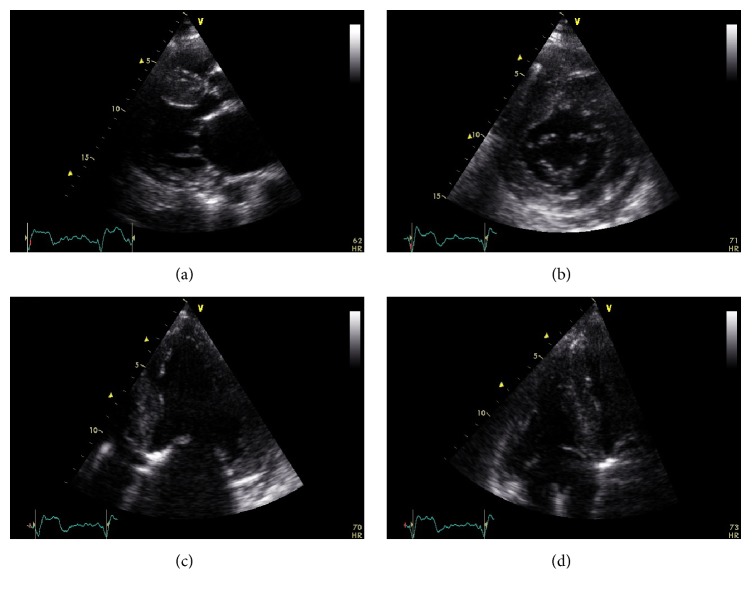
Echocardiographic images from a patient with advanced Anderson-Fabry disease and end-stage renal failure. Parasternal long axis (a), short axis (b), and apical four-chamber view (c). There is left ventricular hypertrophy, more pronounced at the interventricular septum. There is also thickening of the right ventricular free wall apparent at the modified apical four-chamber view (d).
